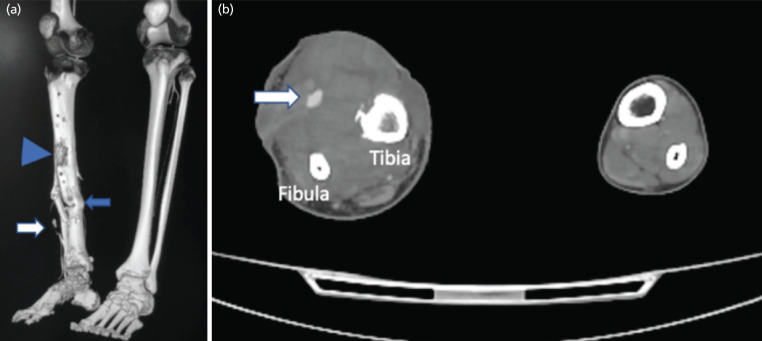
(a) CT angiography right lower limb. The white arrow showing the location of aneurysm. The blue arrow is the docking site. The arrow head is the new regenerated bone. (b) Image showing the aneurysm (white arrow) has been pushed more anteriorly by the hematoma.
